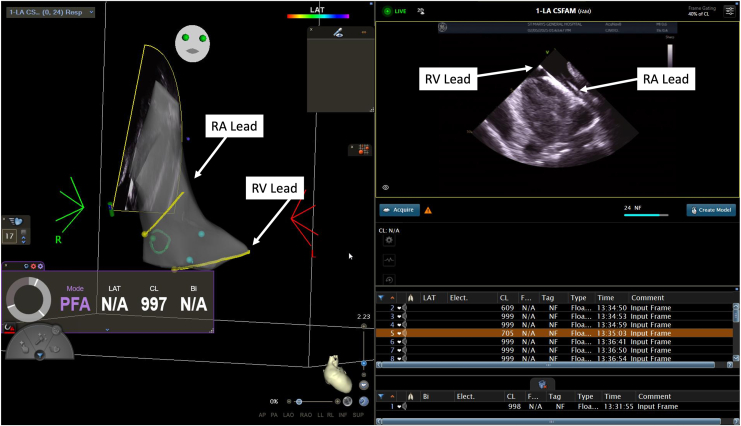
Intracardiac echocardiography (ICE) visualization of intracardiac pacemaker leads: ICE catheter in the right atrium with a posterior tilt and right steer to visualize 2 intracardiac pacemaker leads in the superior vena cava. Upper yellow line indicates right atrial (RA) lead; bottom yellow line indicates right ventricular (RV) lead; green circle indicates coronary sinus os; light blue dots indicate tricuspid annulus. Legend: upper right yellow line = right atrial (RA) lead, bottom right yellow line – right ventricular (RV) lead, green circle – coronary sinus os, light blue dots – tricuspid annulus
